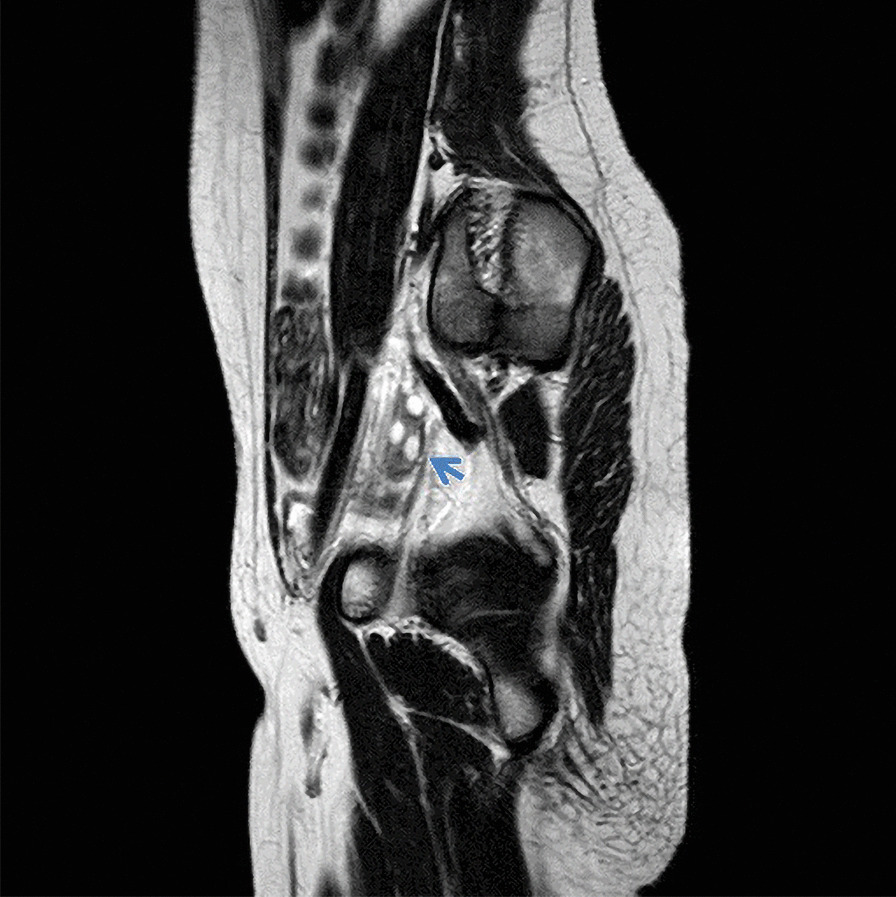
Nuclear magnetic resonance imaging. Paramedian sagittal image enhanced on the left in T2. Left ovary (arrow) is highlighted
